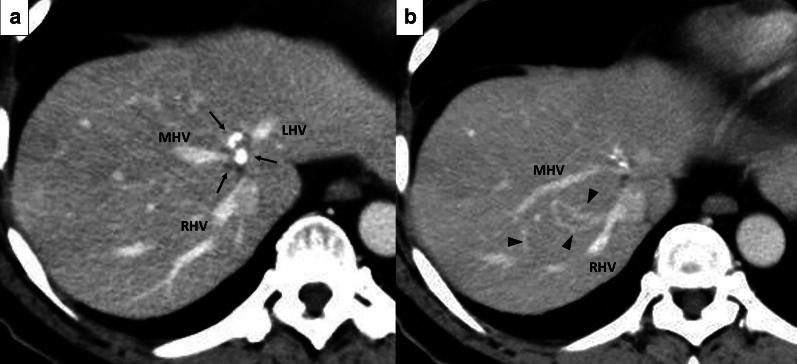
Preoperative computed tomography images (Case 1). a A metastatic tumor (arrows) involved the root of the MHV and left hepatic vein (LHV). The root of the RHV was intact. b Veno-venous communications between the MHV and RHV (arrowheads) that had developed as a result of MHV obstruction by tumor invasion
